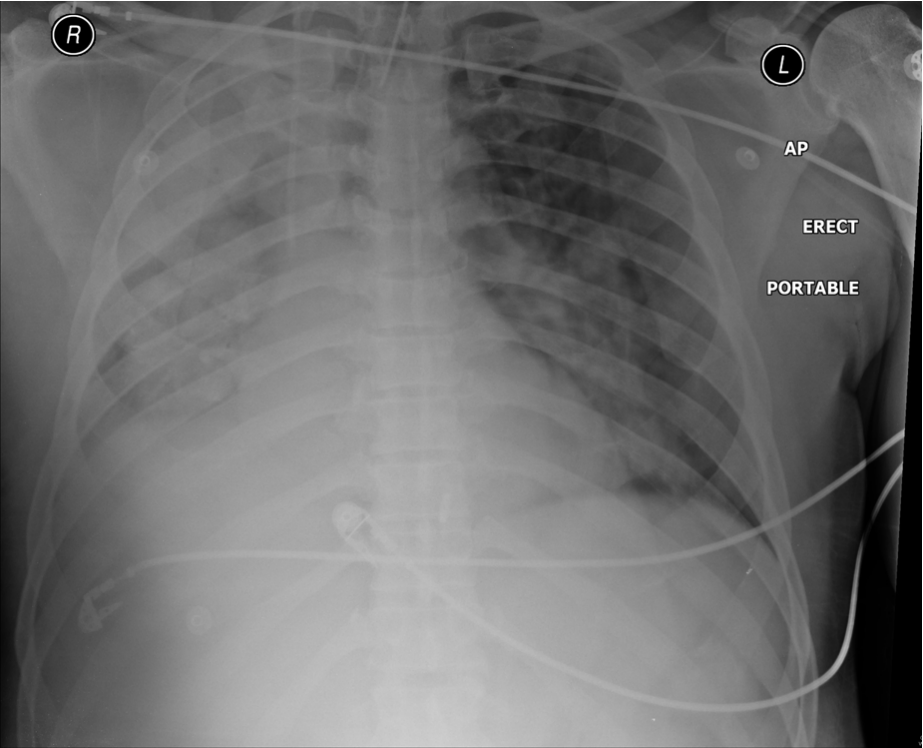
Chest radiograph on day five.
